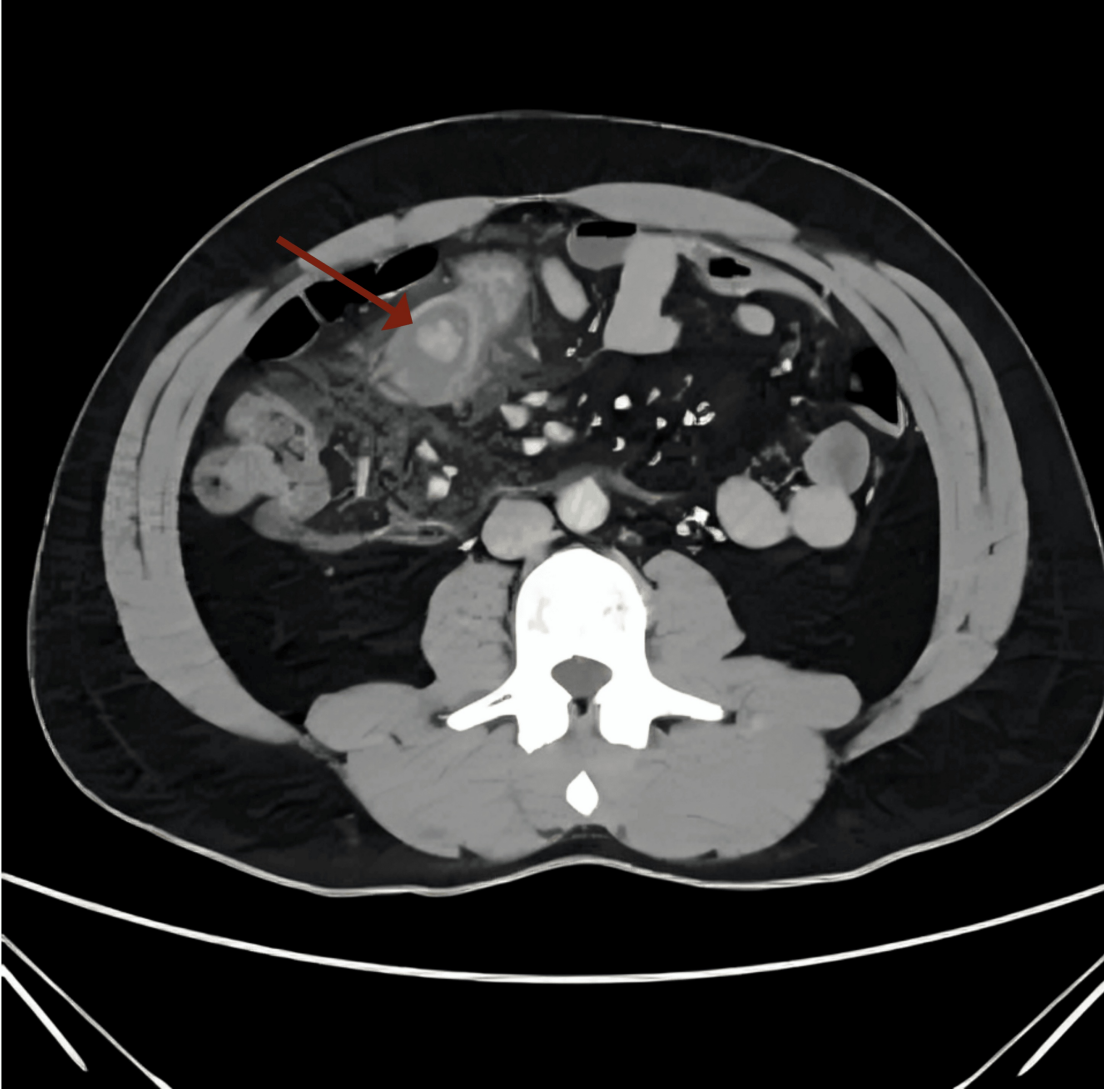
A computed tomography (CT) scan with intravenous contrast showing a suspected micro-perforation in the right upper quadrant of the abdomen
